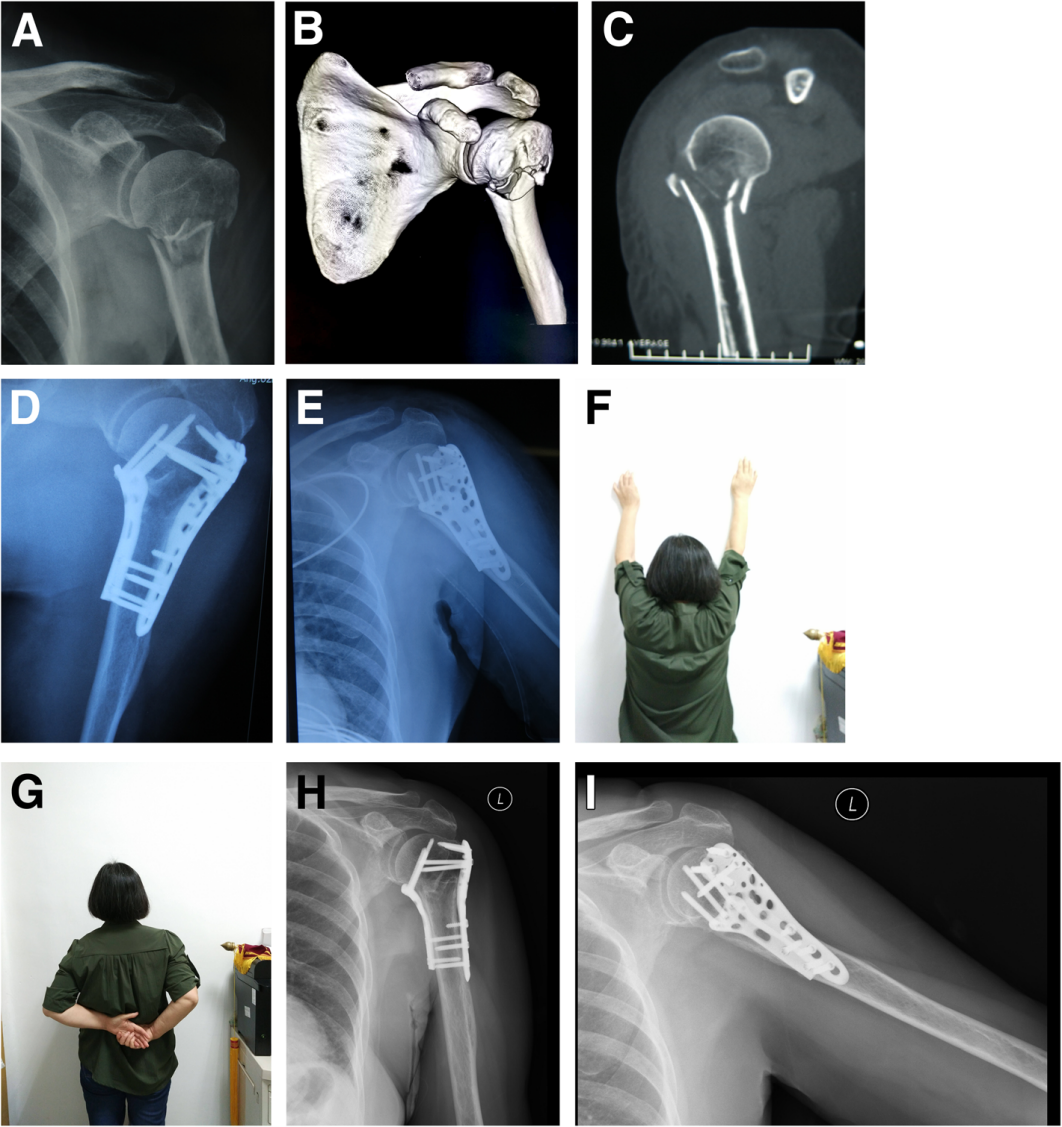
Case 1. A 62-year-old woman. AP X-ray (a) and 3-D CT (b, c) of the patient’s left shoulder immediately after injury demonstrating a fracture of the proximal humerus. Immediate postoperative anteroposterior (d) and lateral (e) radiographs. Clinical (f, g) and radiological (h, i) results 12 months postoperatively (Constant score 84)
